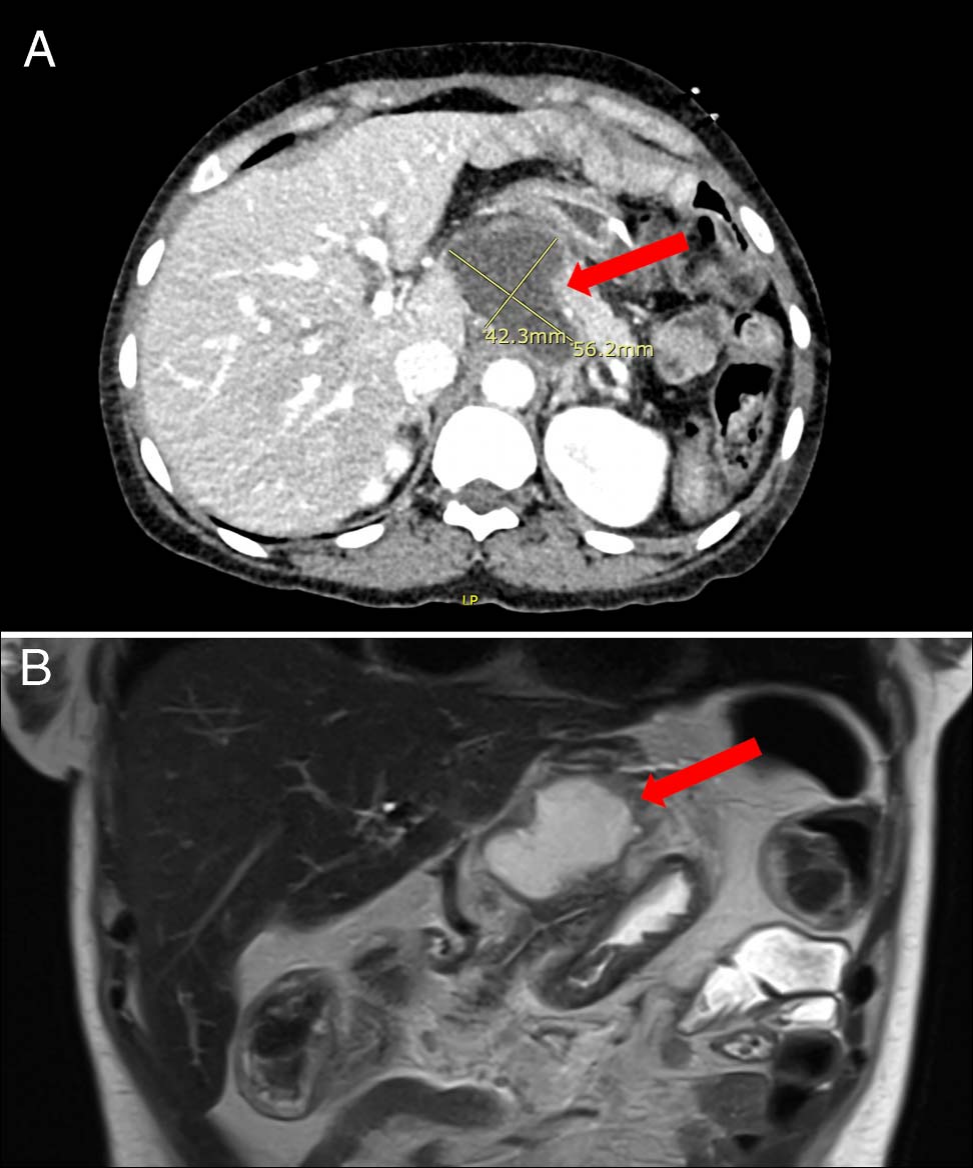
(A) CT scan showing a peripancreatic fluid collection measuring 5.6 × 4.2 × 3.3 cm with mild peripancreatic fat stranding. (B) Magnetic Resonance Imaging/Magnetic Resonance Cholangiopancreatography confirming a well-defined peripancreatic fluid collection measuring 6 × 4.2 × 4.8 cm adjacent to the celiac axis.
